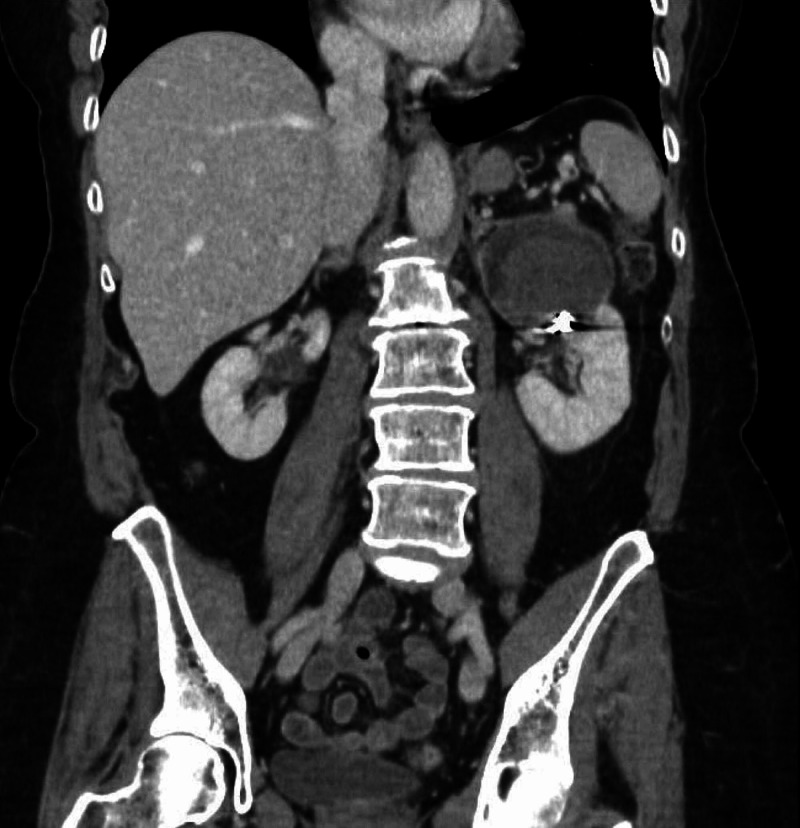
Coronal reconstruction of nephrogenic phase contrast-enhanced CT abdomen and pelvis one year following embolization demonstrate partial resorption of the left upper pole spontaneous renal hemorrhage. No renal mass is identified.
